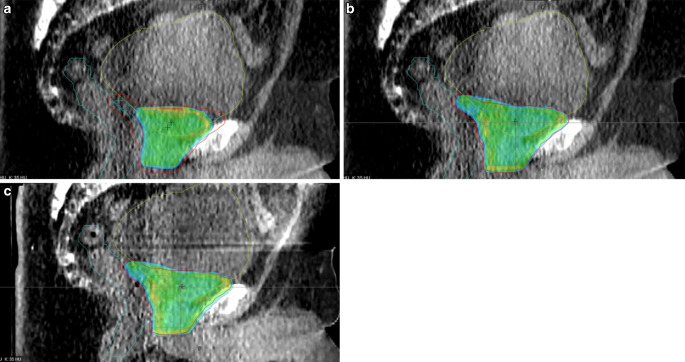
Visualization of the 95% isodose with colorwash towards 105% of the prescribed dose reveals a the scheduled plan (SCH) depicting a planning target volume (PTV, red) barely covered potentially due to fluctuations in bladder volume (yellow), while b the adapted plan (ADP) showcases a newly optimized dose distribution with improved coverage. c Dose reconstructed on the postadaptive verification CBCT
